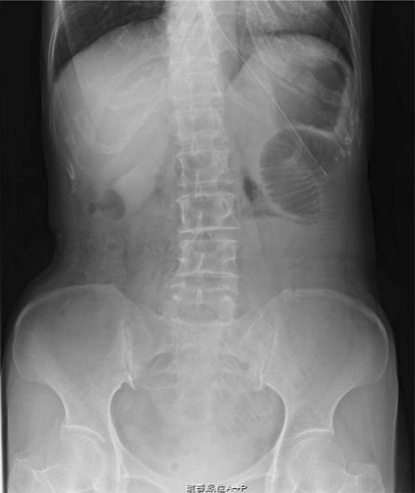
Plain abdominal radiograph. The air-fluid levels in the* left upper quadrant*
